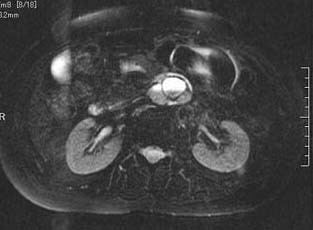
T2-weighted magnetic resonance images revealed a high intensity tumor those signal intensity was greater than that of the liver with central higher signal intensity.
